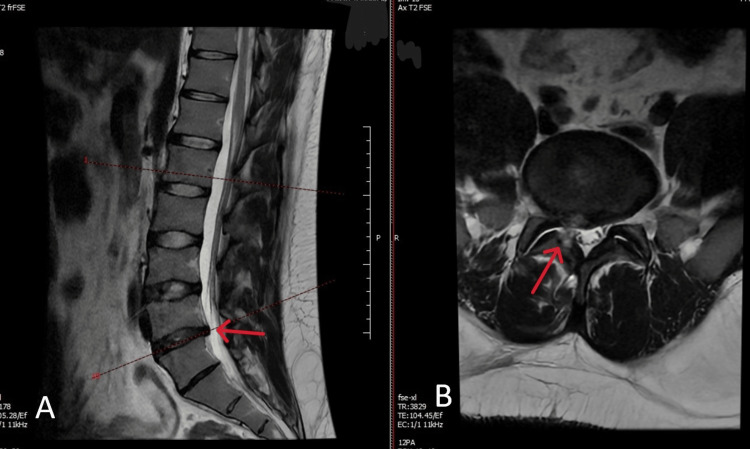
Early postoperative lumbar MRI. (A) Sagittal T2-weighted MRI showing adequate decompression at L4–L5 (red arrow). (B) Axial T2-weighted MRI demonstrating complete removal of the herniated fragment and relief of right L5 nerve root compression (red arrow).
